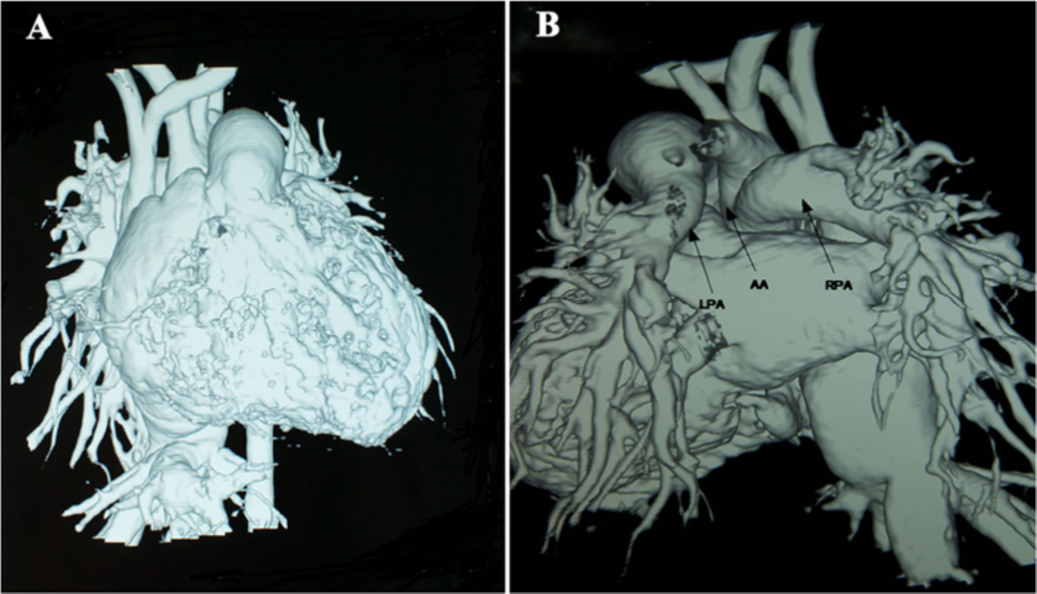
Preoperative CT angiography of Patient 2 identified the anomalous origin of the right pulmonary artery from the ascending aorta; a the antero-posterior VR. b the postero-anterior VR. AA: ascending aorta, LPA: left pulmonary artery, RPA: right pulmonary artery
